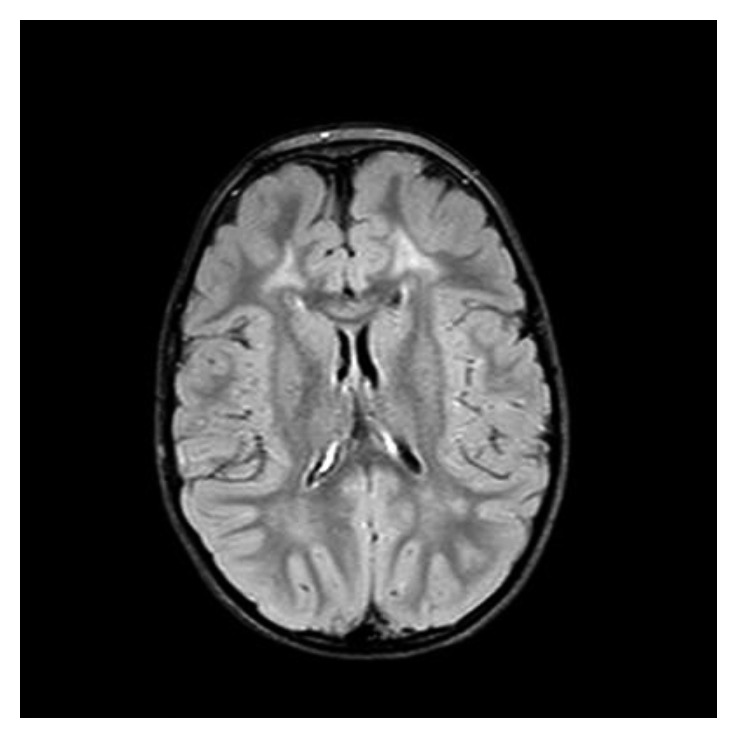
Flair axial MR image of the brain showing high-intensity lesions in the deep white matter of the frontal lobes and milder-intensity lesions in the deep white matter of the posterior parietal lobes and the corpus callosum.
